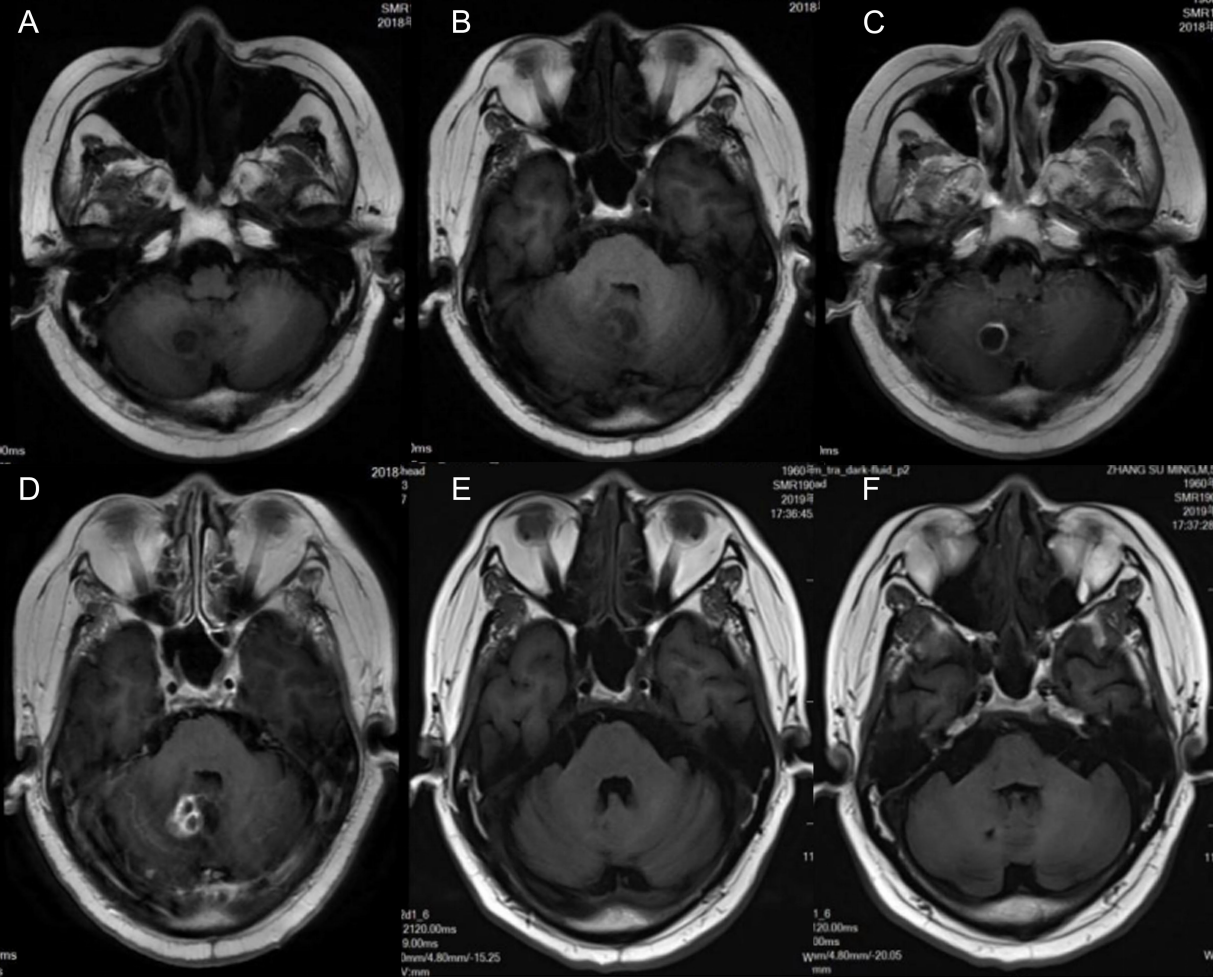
Magnetic resonance imaging (MRI) of the patient shows cerebellar vermis and right cerebellar brain abscess with ring contrast enhancement and peripheral edema. (A,B) T1-weighted imaging. (C,D) Axial and sagittal T1 contrast enhancement imaging. (E,F) T1-weighted imaging 4 months after treatment; the lesion was reduced markedly.
